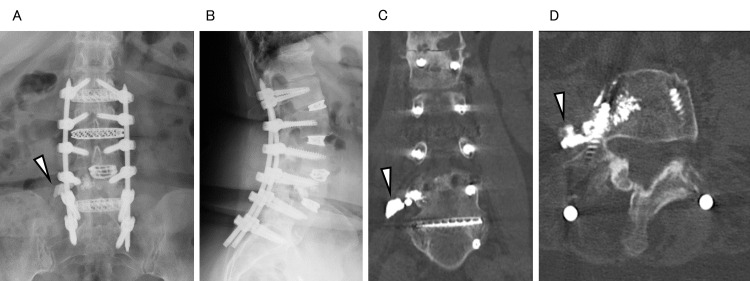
Preoperative images: (A) anteroposterior (AP) X-ray; (B) lateral X-ray; (C) coronal computed tomography (CT) image; (D) axial CT image (arrowheads indicating the leaked cement).
